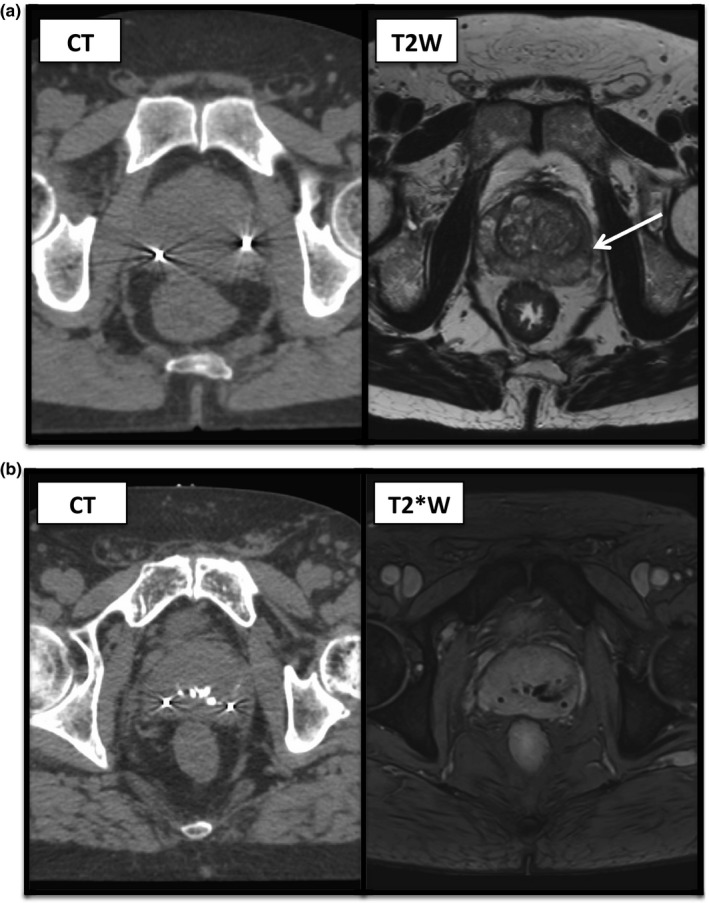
(a) Corresponding CT (left) and T2W (right) images for a patient showing the appearance of a fiducial marker on standard T2W imaging, as indicated by the arrow. The second fiducial marker visible on CT imaging could not be identified on T2W images here. (b) Corresponding CT (left) and T2*W (right) images for a patients showing two fiducials with surrounding artifact on CT images and central calcifications, all showing as signal loss on T2*W imaging.
